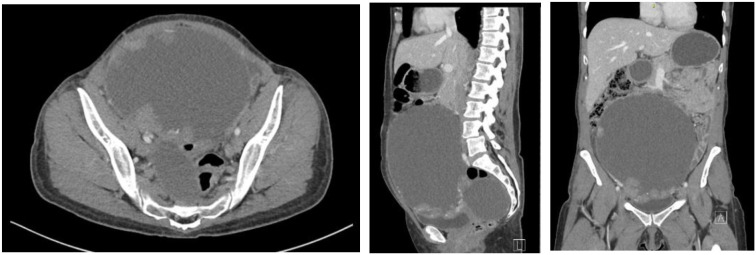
CT AP with contrast 2/4/2021: 18.4cm cystic mass arising from the right adnexa, with an additional 8.8cm cystic lesion in the posterior right pelvis.
